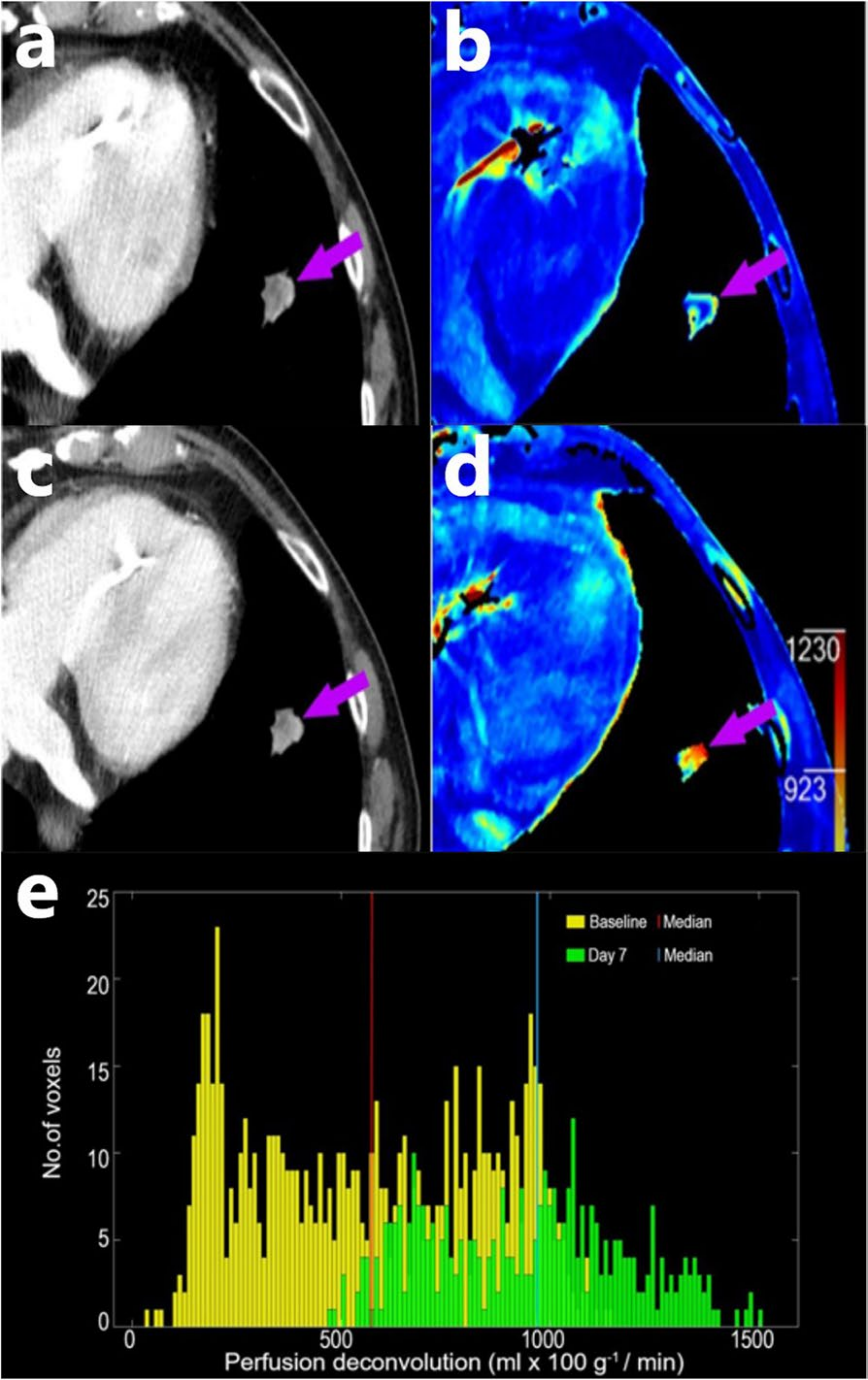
A patient with NSCLC in the left lung on contrast-enhanced CT at baseline (a) and seven days after baseline (b); and the corresponding DCE-CT BF_decon_ map at baseline (c) and seven days after baseline (d). The BF_decon_ histogram of the tumor depicts an increase of 69% in median BF_decon_ values from baseline (573 ml x min^−1^ × 100 g^−1^) to day seven (968 ml x min^−1^ × 100 g^−1^). BF_decon_ = Blood flow deconvolution, DCE-CT = Dynamic contrast enhanced computed tomography, NSCLC = Non-small cell lung cancer
